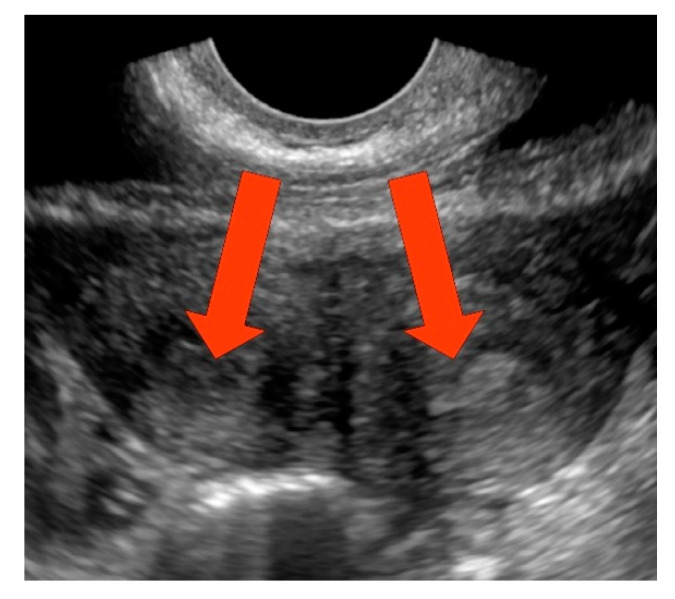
Sonogram of a 13-year-old patient imaging two uterine bodies with endometrium. There was no blood in the uterus.
